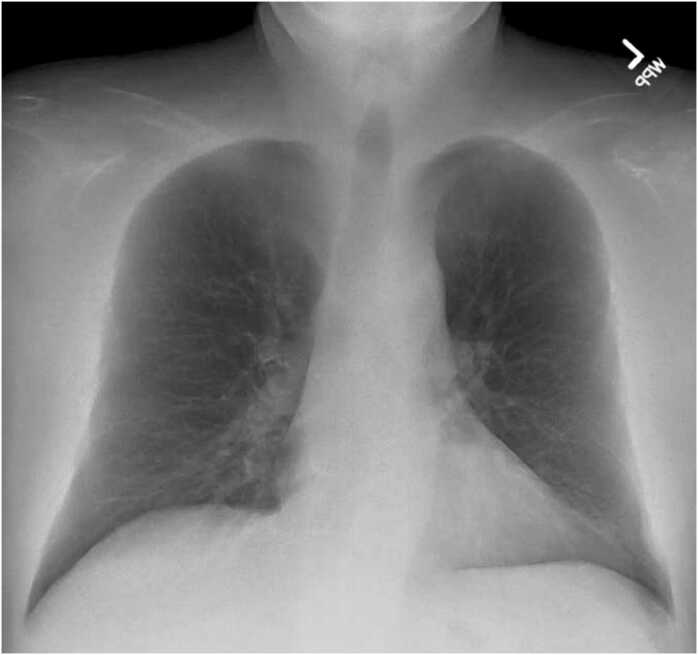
Chest X-ray: posteroanterior view. Mild bronchial wall thickening.
